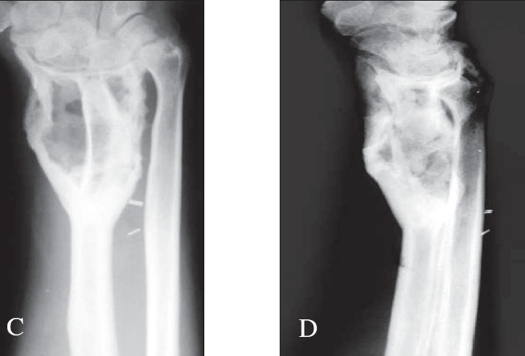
X-rays (A.P. and lateral) of the same patient showing good consolidation
